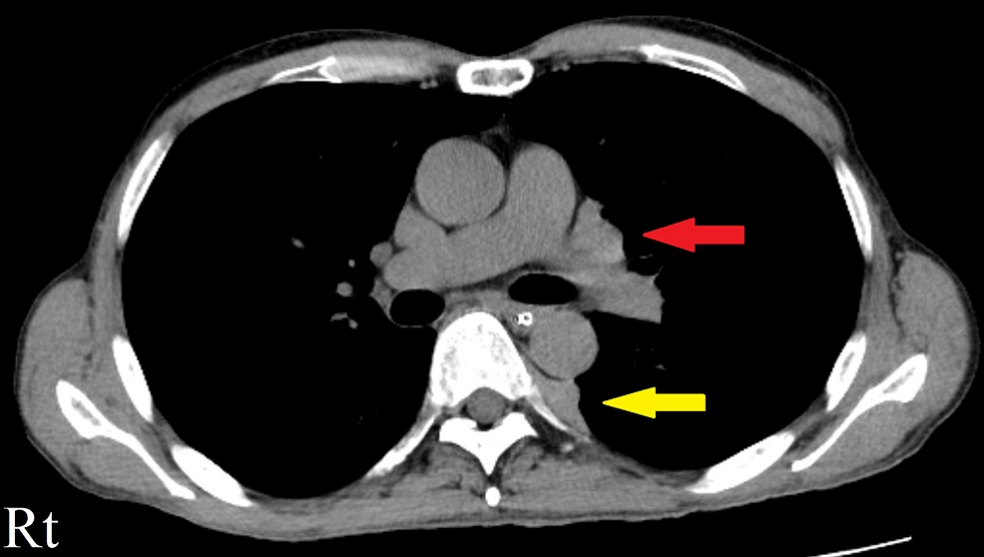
Transverse sections on CT (soft tissue window setting) demonstrating the metastatic thymoma in the patient in Case 1. A slight reduction in the size of the invasive thymoma metastasis in the mediastinal region (red arrow) and lateral pleura (yellow arrow) can be seen. CT, computed tomography
